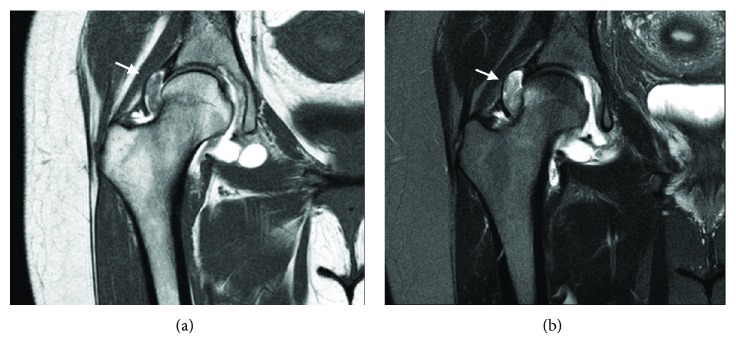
(a) T1 coronal magnetic resonance image showing nodular intra-articular mass with low signal intensity (arrow). (b) T2 fat-saturated coronal magnetic resonance image showing nodular intra-articular mass with high signal intensity (arrow).
